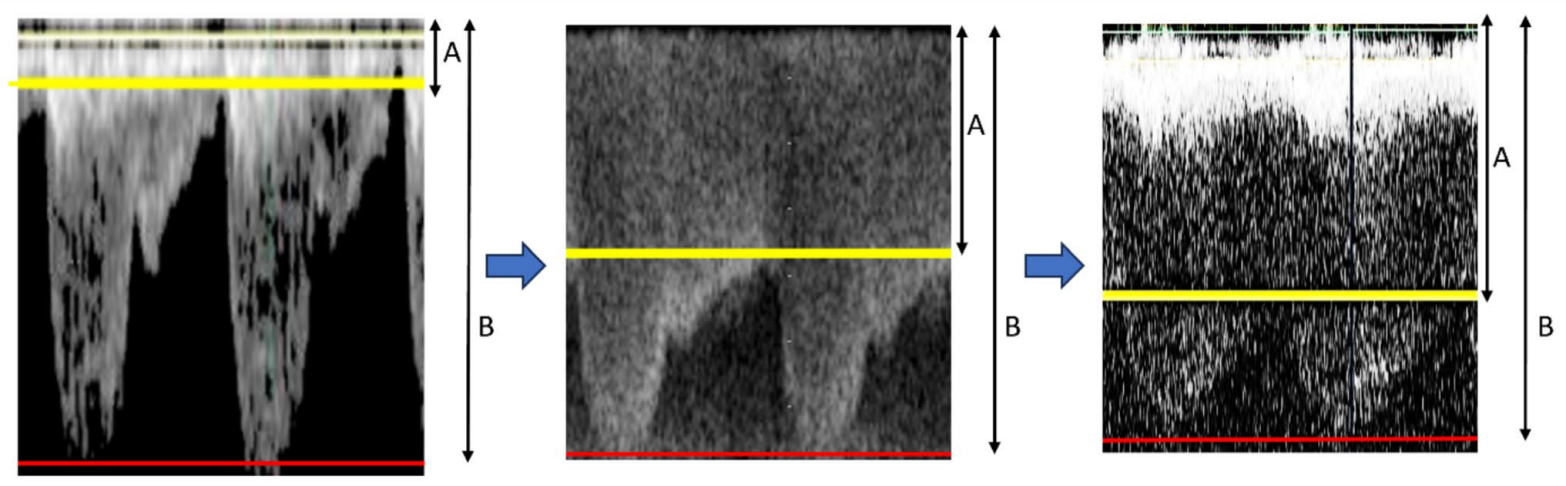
Intraoperative continuous-wave Doppler echocardiographic images across banding site of pulmonary artery. A (from top to yellow line) is nadir flow velocity. B (from top to red line) is peak flow velocity. Velocity ratio (A divided by B) increases with tighter banding. At the appropriate band tightness, the echocardiographic flow image depicts excessive pulmonary blood flow (left image), appropriate pulmonary blood flow pattern (middle image), or insufficient pulmonary blood flow (right image)
